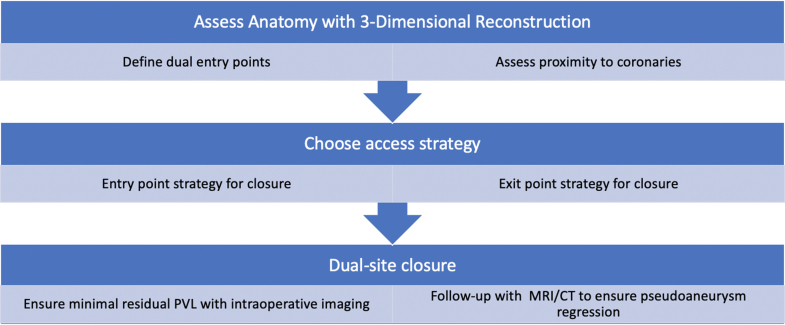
Algorithm for the Approach to Complex Paravalvular Leak With an Aneurysmal Tract CT = computed tomography; MRI = magnetic resonance imaging; PVL = paravalvular leak.
